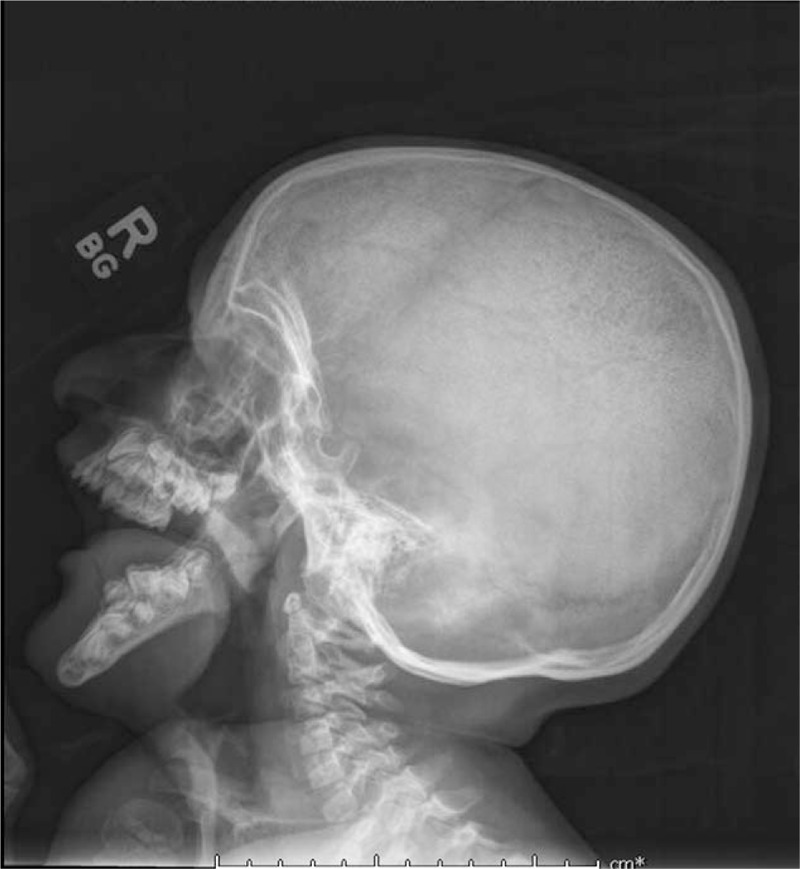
Lateral skull X-ray at age 5.
